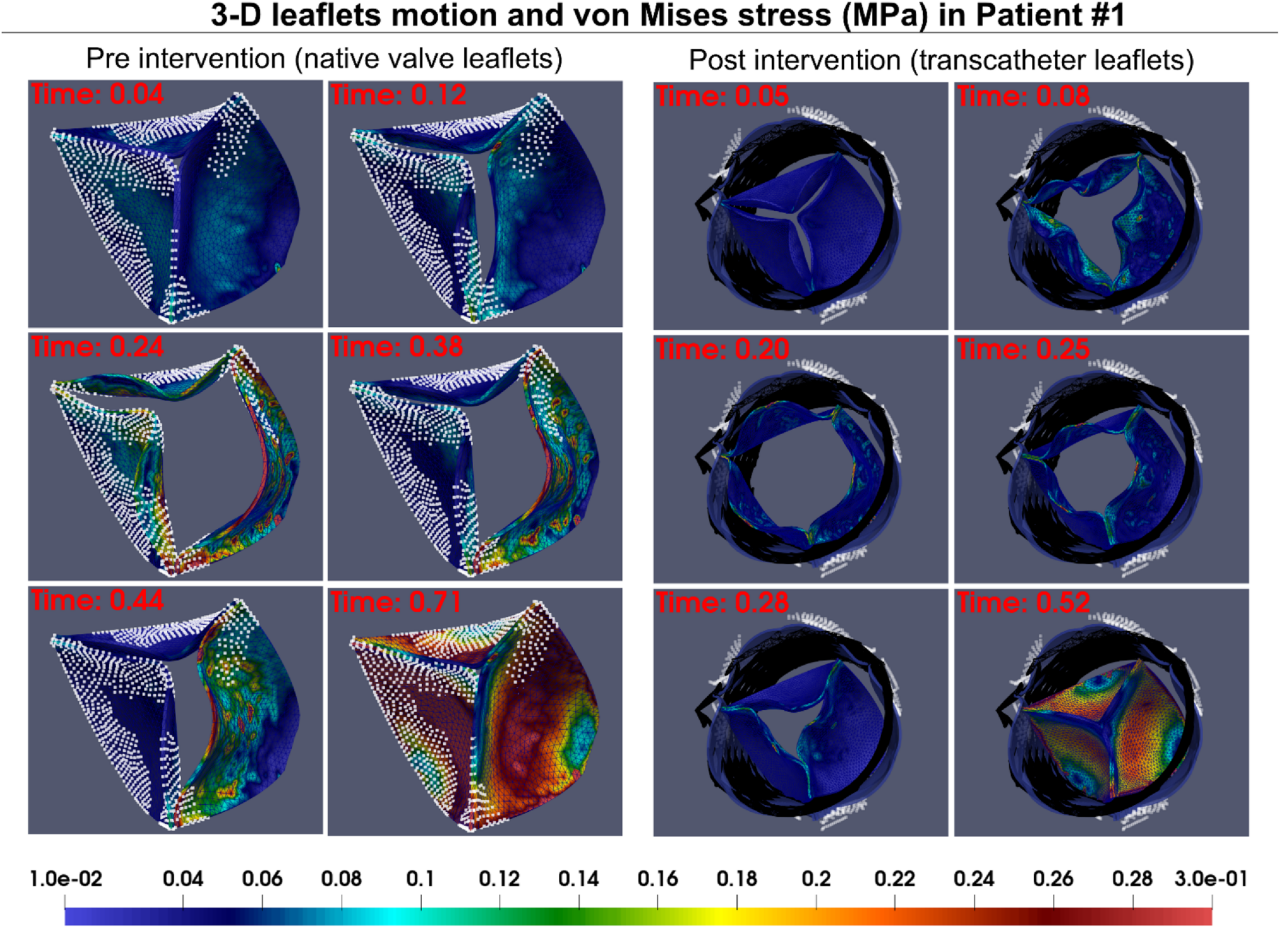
3D motion and 3D distribution contours of von Mises stress in patient #1 at six time points throughout the cardiac cycle in both pre- and post-intervention states. Using our framework, we estimated 3D deformation of aortic valve leaflet during full cardiac cycle as well as the von Mises stress distribution. The regions covered with white points are representing the calcified areas visualized manually by using multi-slice CT images.
